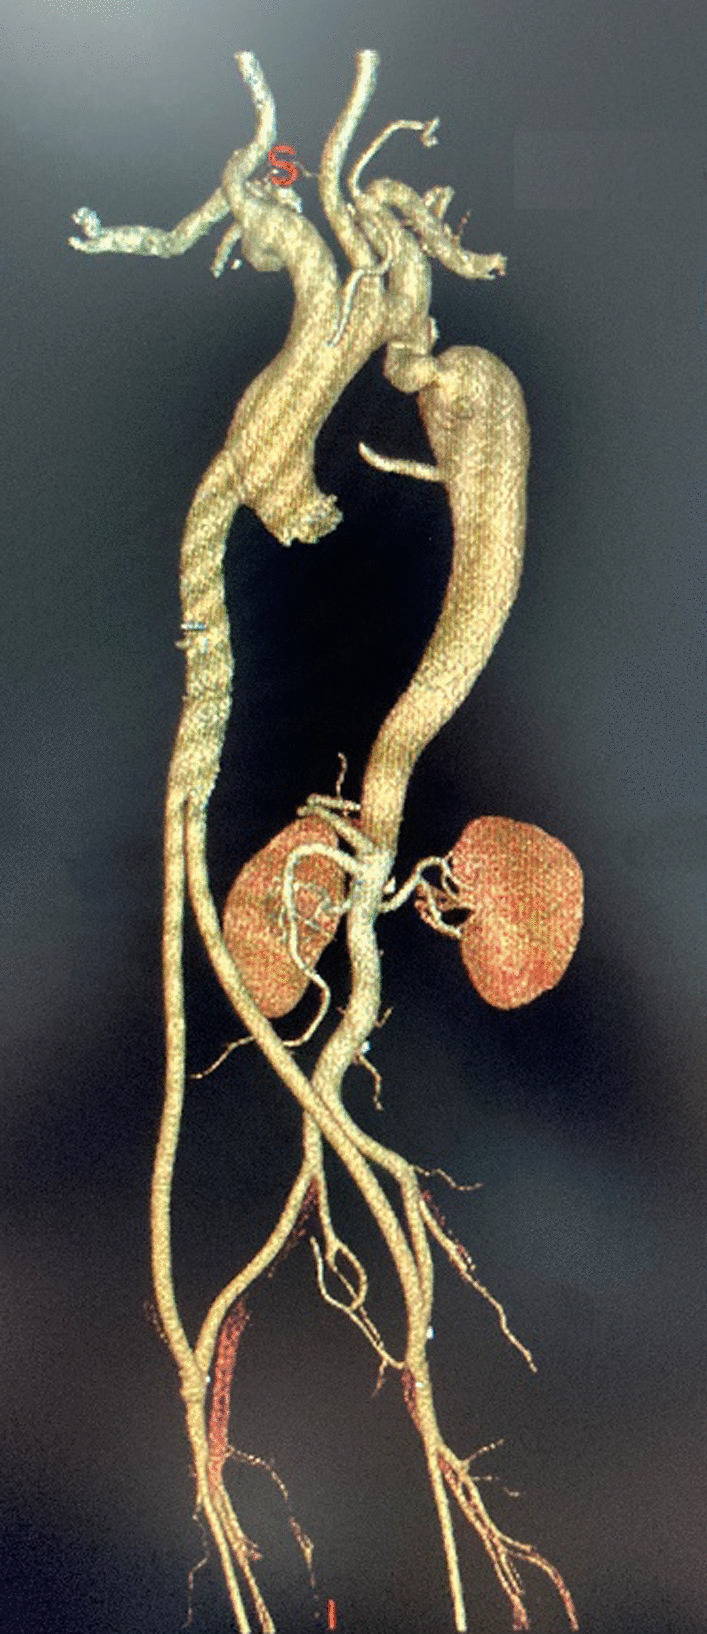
Postoperative CTA of AFB
